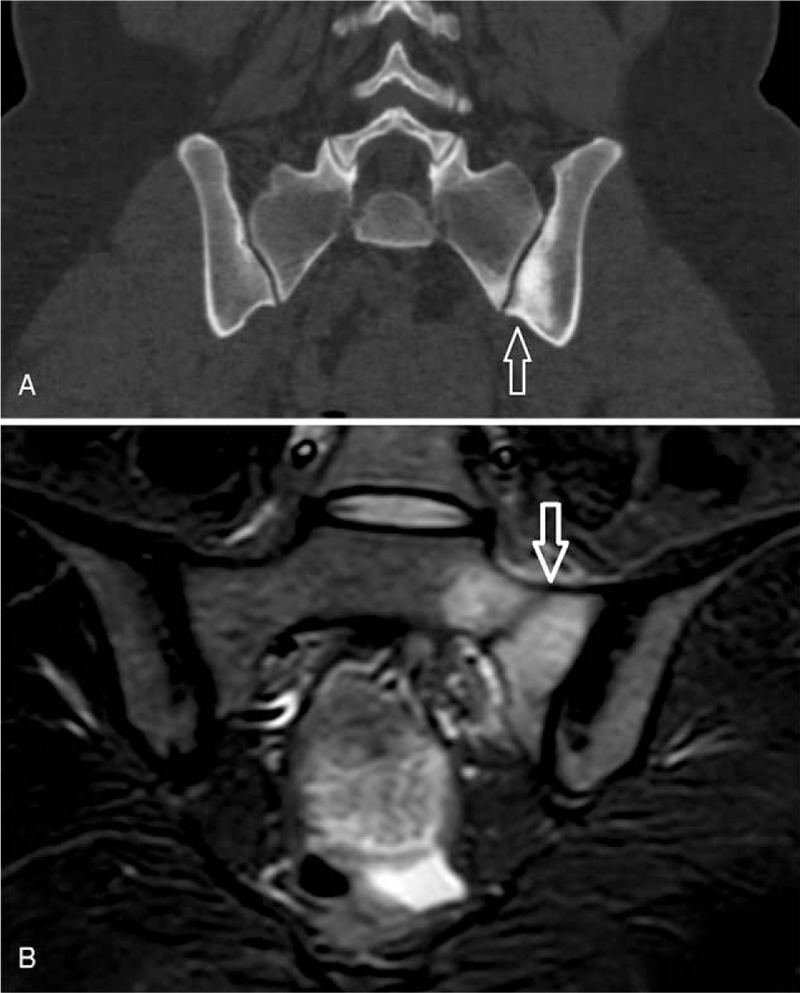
CT scan (A) and T2-STIR MRI (B) images showing respectively a condensation of the left sacroiliac joint (arrow) and a hypointense oblique fracture line surrounded by an area of edema on the left side of the sacrum (arrow). CT = computed tomography, T2-STIR MRI = T2-weighted short-tau inversion recovery magnetic resonance imaging.
